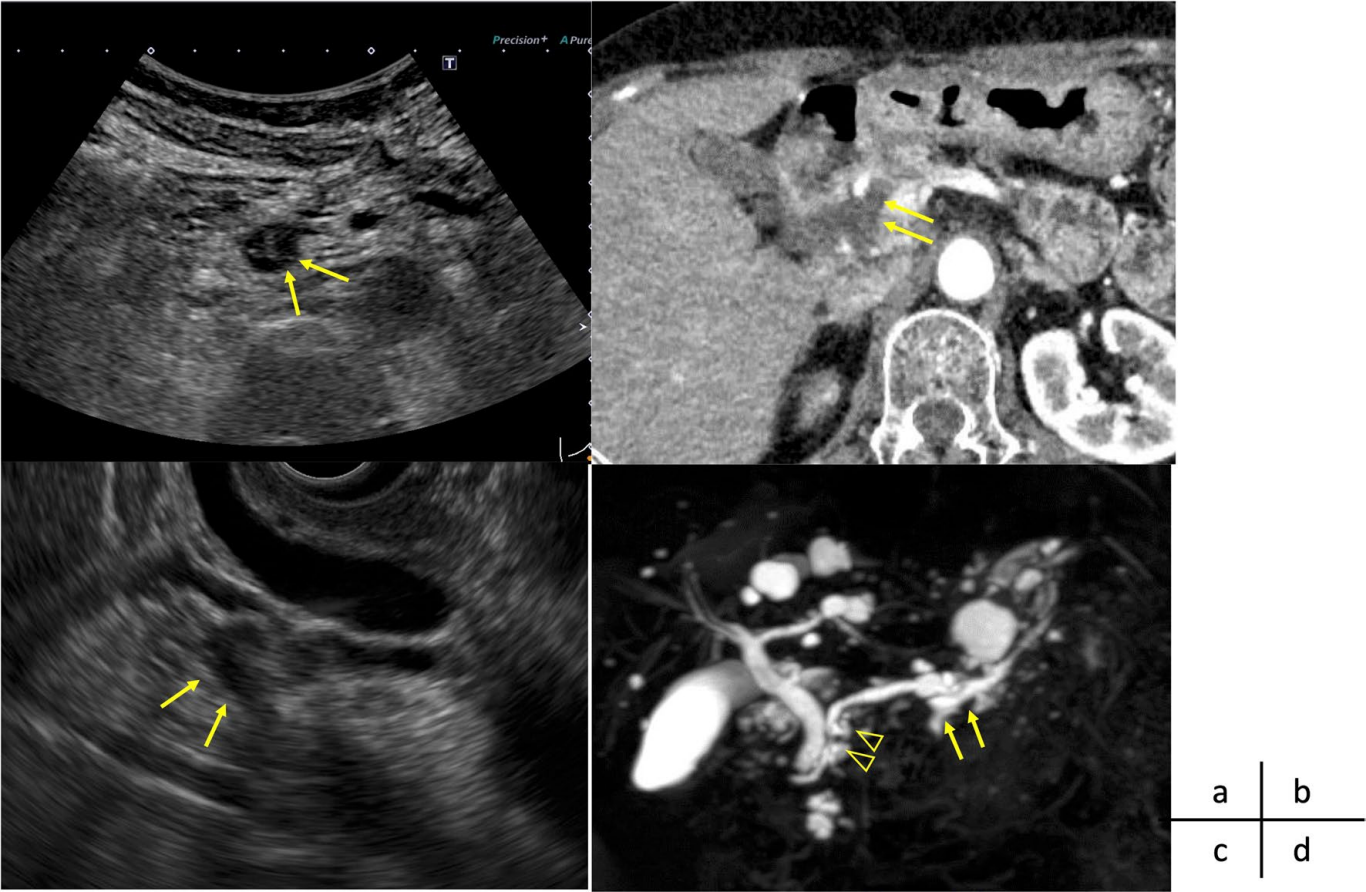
Preoperative imaging findings. a Abdominal ultrasonography. An intraluminal nodule is suspected in the cystic lesion at the uncinate process (arrow). b Computed tomography in the arterial phase. A 16-mm-diameter cystic lesion is detected in the uncinate process (arrow), and a solid portion in the cyst is not evident. c Endoscopic ultrasonography. The cystic lesion shows a thickened wall, and the intramural echoic shadow is unclear; however, intramural nodules and mass are not detected (arrow). d Magnetic resonance cholangiography. Main pancreatic duct stricture (triangle) at the pancreatic duct is newly detected. The cystic lesion in the pancreatic body has not changed since the previous imaging study (arrow)
